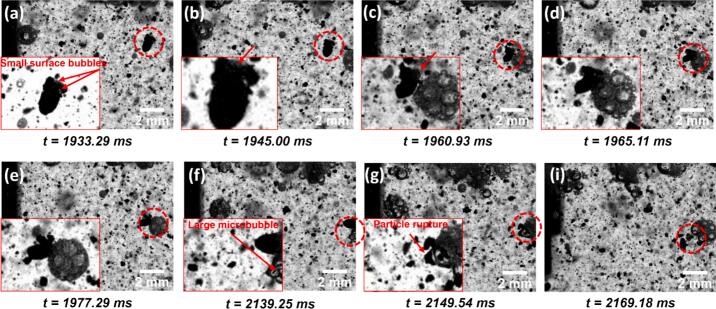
A deagglomeration event captured on the side of sonotrode in TX-100 solution during UST. The inset of each figure with adjusted brightness and contrast shows the zoomed in image of the MWCNT agglomerate labelled by red circle. The red arrow in (a-c) indicates the position of surface bubbles observed on the MWCNT agglomerate. The red arrow in (f) indicate the microbubble that caused final rupture of the agglomerate (g). (Supplementary Video 3 (SV3)). Time values represent the elapsed time after the onset of ultrasonication. (For interpretation of the references to colour in this figure legend, the reader is referred to the web version of this article.)
